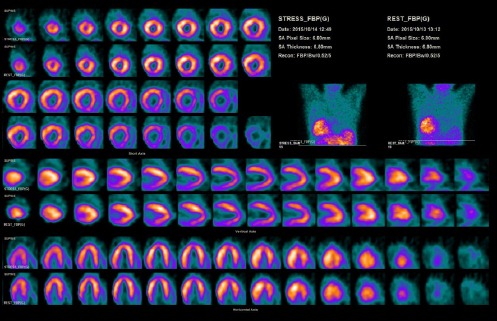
^99m^ Tc-MIBI SPECT images reconstructed with conventional processing, showing the right ventricle where the left ventricle is usually seen. Note the myocardial walls with a different localization
